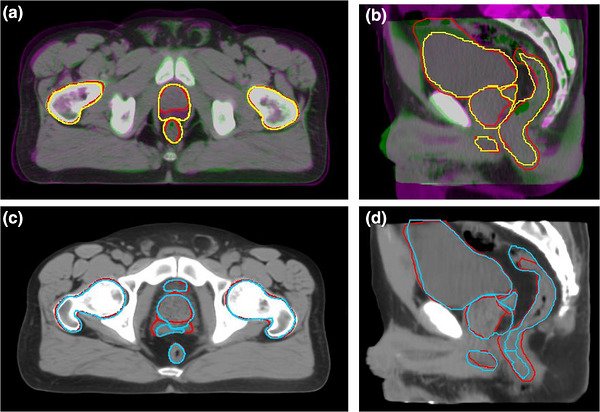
Representative pelvic contour overlays for a prostate patient, shown in axial (left) and sagittal (right column) views. Top row (a, b): CPAI contours generated on the reference CT (yellow) versus on the sCT (red), displayed on the rigidly registered CT (magenta)‐sCT (green) fusion to compare both sets on common anatomy. This corresponds to the geometric agreement quantified in Figure 6. Bottom row (c, d): CPAI (red) versus TheraPanacea (light blue) contours, both on the sCT. Structures shown: femoral heads, bladder, penile bulb, prostate, seminal vesicles, and rectum.
